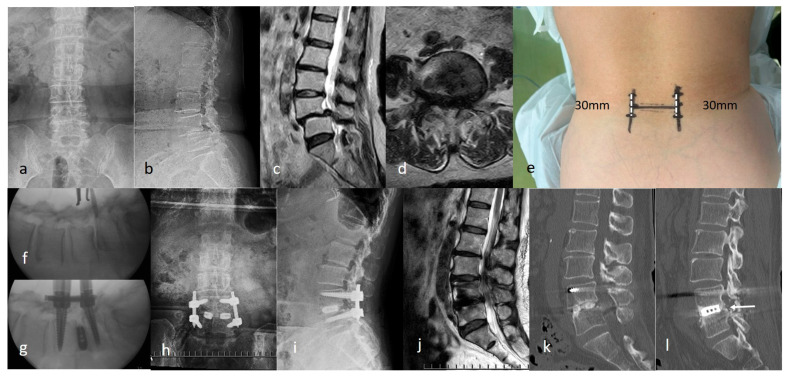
Case of grade 2 spondylolisthesis at L4/5. (a,b) Preoperative radiograph. (c,d) Preoperative magnetic resonance images revealing spinal canal stenosis at L4/5. (e) The procedure was performed through 30-mm bilateral incisions. (f,g) Reduction of the slippage using pedicle screw devices. (h,i) Postoperative radiograph. (j,k) Postoperative magnetic resonance and computed tomography images showing a significant reduction in slippage and canal stenosis. (l) Intervertebral bone fusion posterior to the cage (white arrow).
